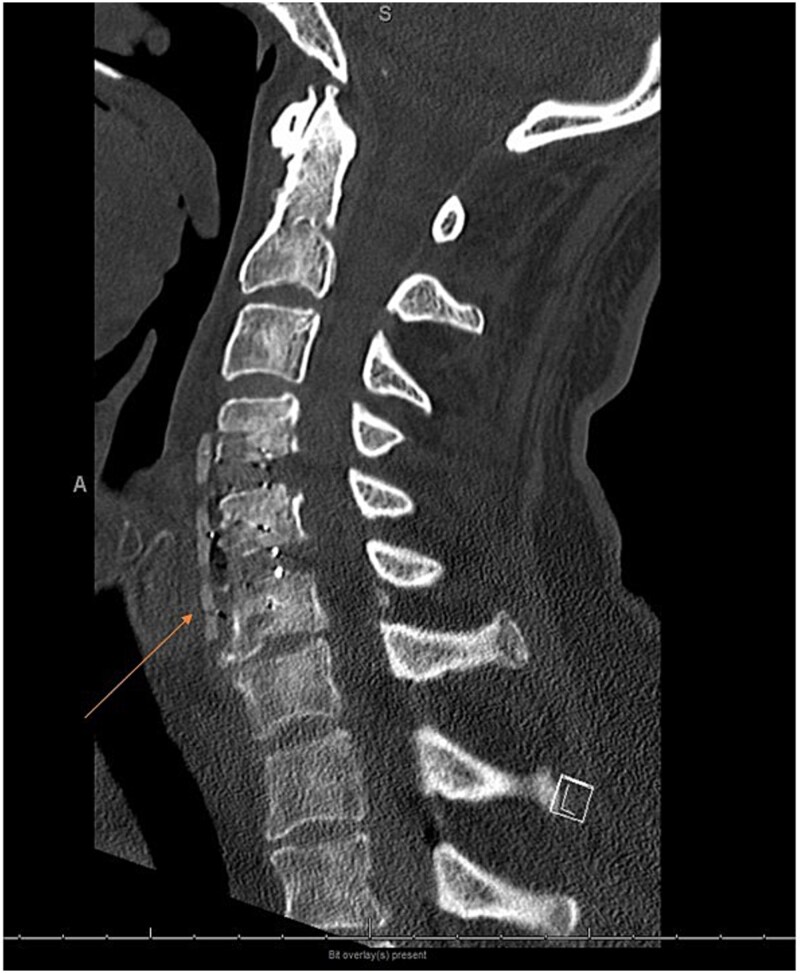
Postoperative sagittal cervical CT scan image sagittal view showing a carbon fiber plate with screws.
